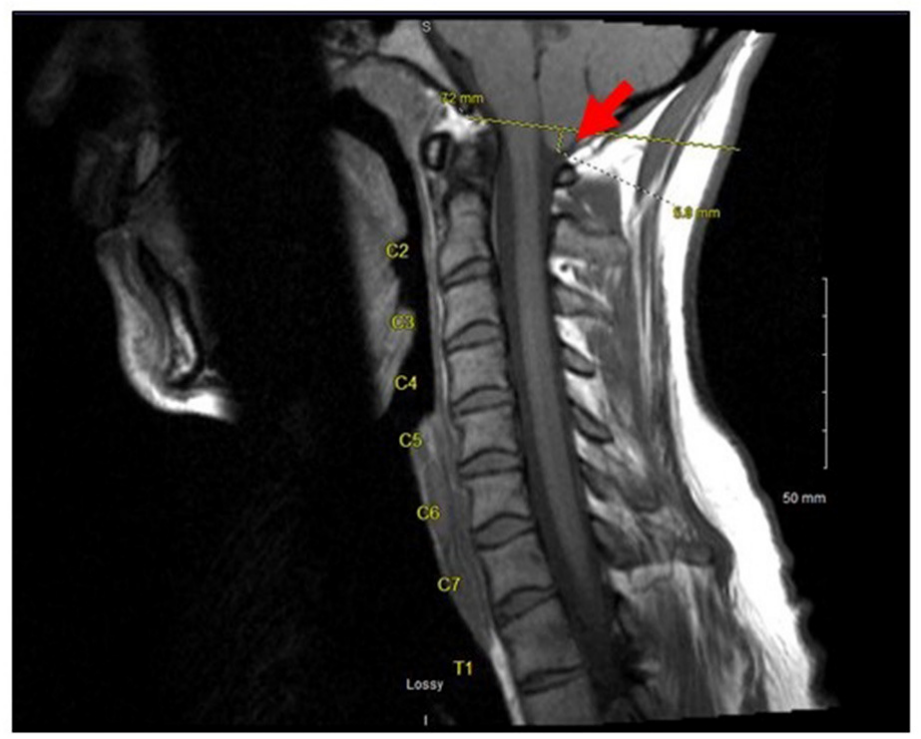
A magnetic resonance imaging of the cervical spine showing a Chiari I malformation (red arrow) with a 5.8 mm displacement of the cerebellar vermis through the foramen magnum.
